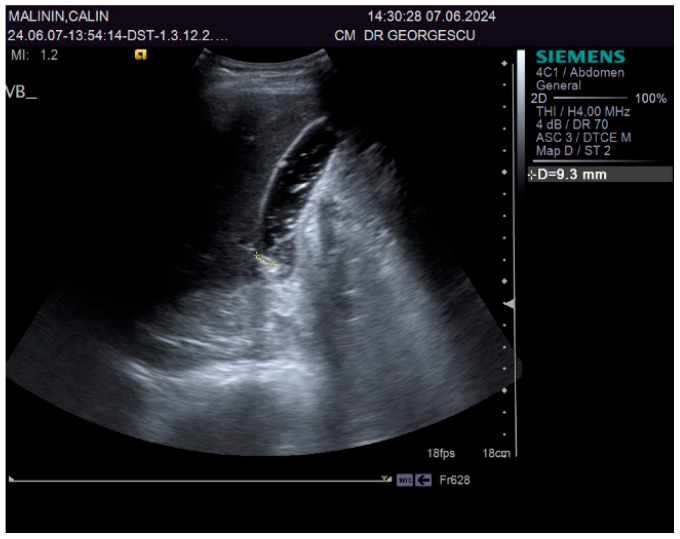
Ultrasound aspect: gallstones.
